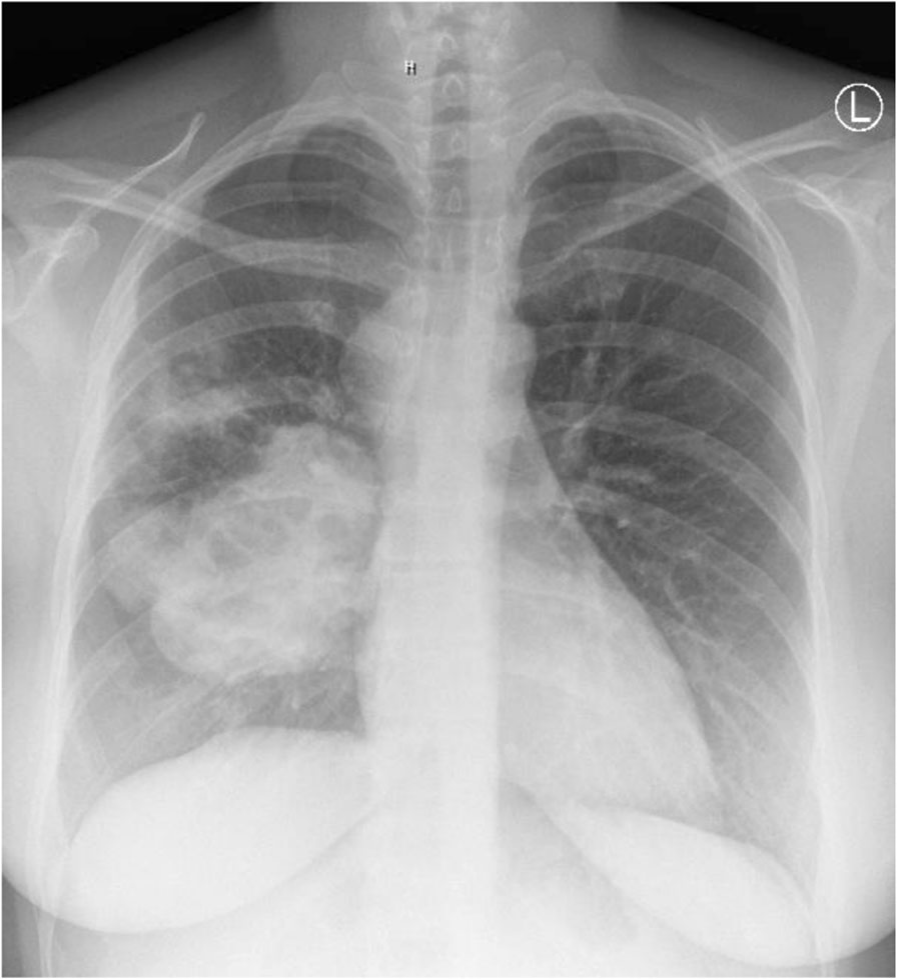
X-ray AP view of thorax showing tumor mass in the right lung
